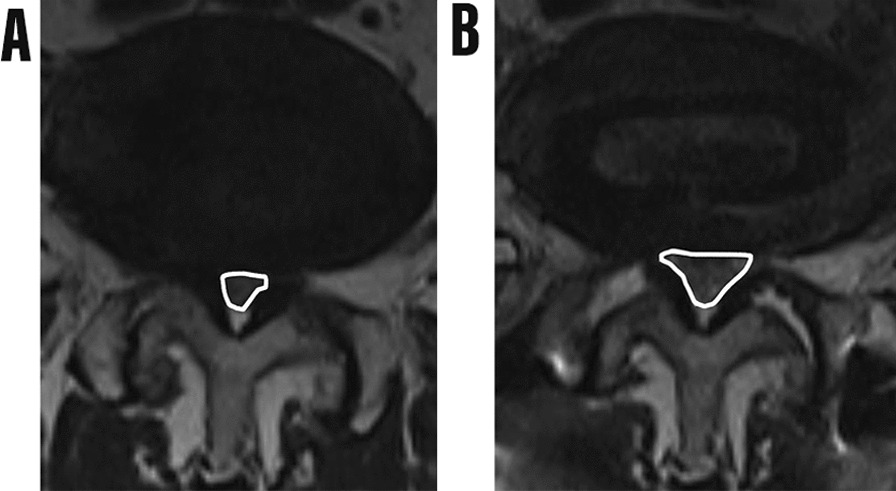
Preoperative and postoperative dural sac CSA was evaluated by MRI imaging. A Preoperative dural sac CSA. B Postoperative dural sac CSA
